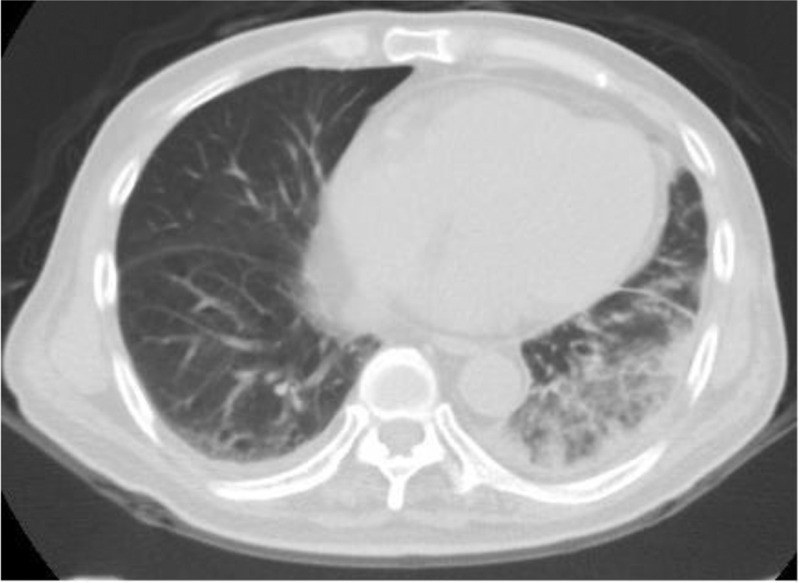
Initial chest computed tomography (CT): left lower lung air-bronchial ground with pleural effusion.
